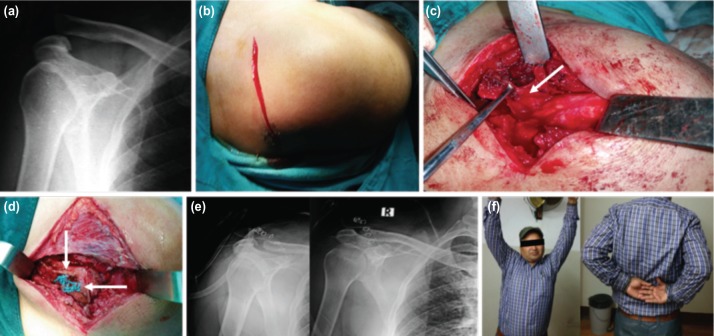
(a) Preoperative antero-posterior radiograph showing the type V acromial-clavicular joint dislocation. (b) Antero-posterior incision on lateral aspect of clavicle. (c) Resection of lateral end of clavicle and separation of coraco-acromial (CA) ligament along with bony fragment from anterior margin of acromion. (d) Two separate knots of non-absorbable Ethibond suture, medial one for the stabilisation of clavicle in reduced position by passing suture through base of coracoid process and lateral one for encroach of the bony CA ligament to lateral end of clavicle. (e) Postoperative radiograph showing the reduced clavicle at the level of acromion. (f) Motion of shoulder joint 3 months after surgery showing mild restriction on involved side.
